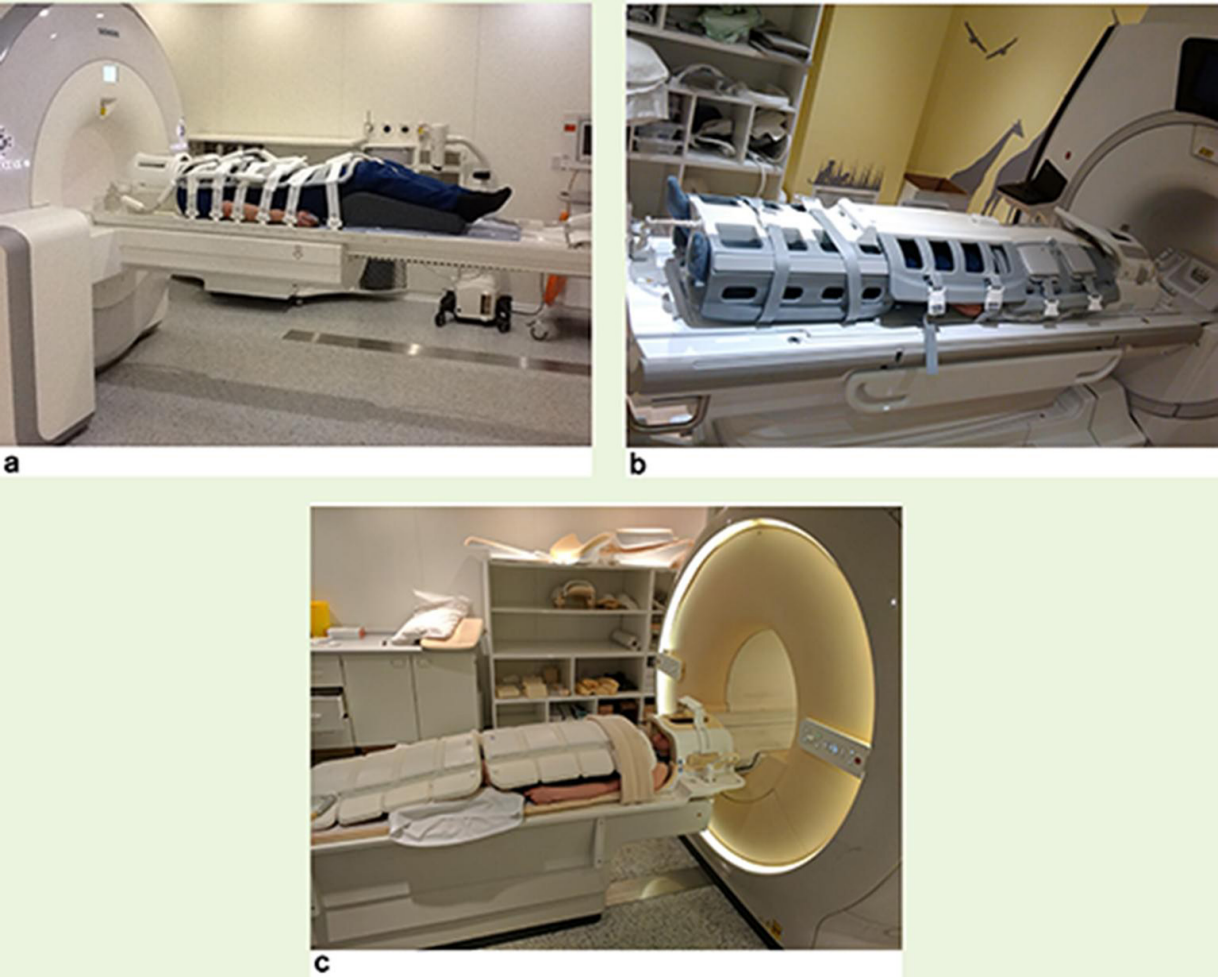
Typical patient set up for a whole body (eyes to thighs) MRI scan (a) Siemens Healthineers (Erlangen, Germany) (b) GE Healthcare (Waukesha, Wisconsin, USA) (c) Philips Healthcare (Koninklijke, The Netherlands).
